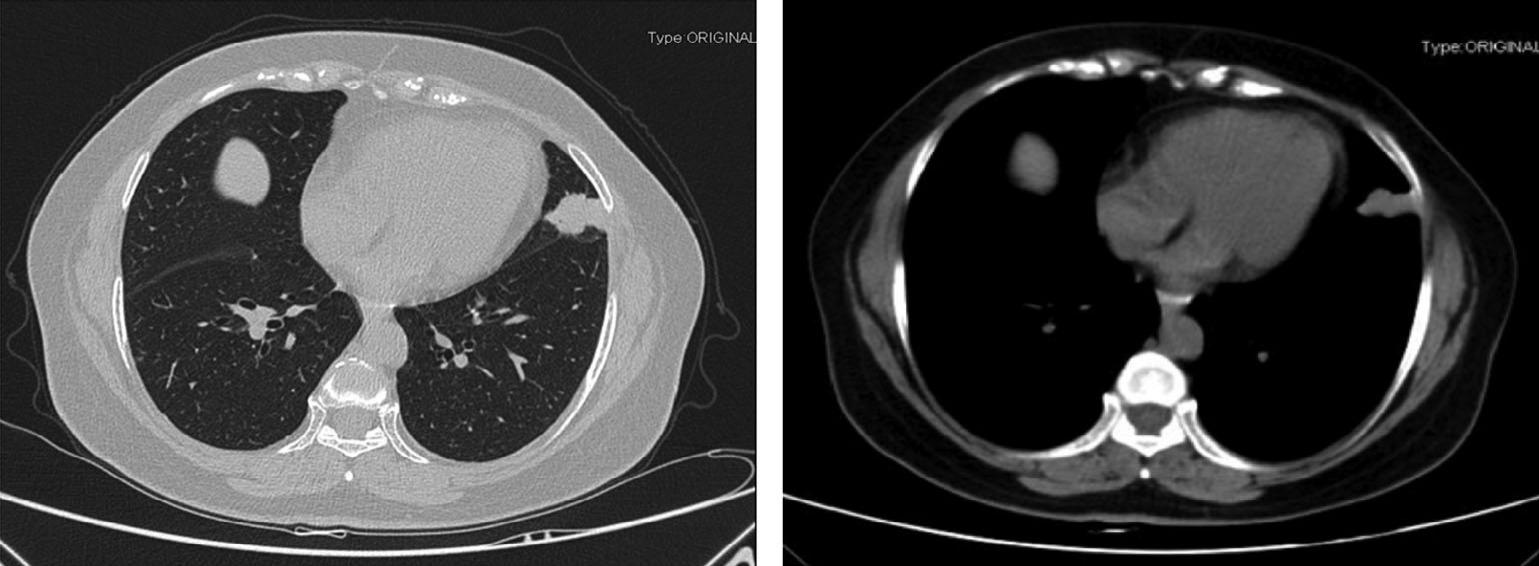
Computed tomography (CT) scan showed the lung tumor mass (2.8 cm × 2.1 cm) in the left upper lobe with pleural invasion.
